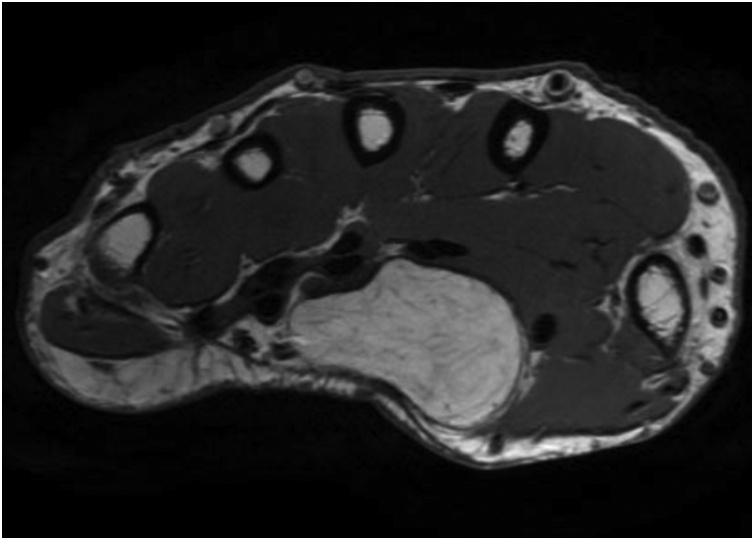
T1-weigthed axial MRI image showing an occupying-space lesion with hypersignal.
